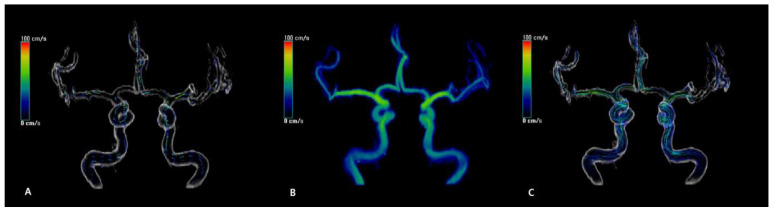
Static captures of four-dimensional flow MRI. Anterior circulation of a 39-year-old woman with normal intracranial arteries. (A) Color-coded three-dimensional (3D) rendering shows velocity vectors, which visualize the direction of blood flow; (B) Color-coded 3D rendering of flow velocity, which displays velocity changes according to the cardiac cycle; (C) Color-coded 3D rendering with streamlines, which represent the path of particles if the particles are released into the velocity field while the field is kept constant.
